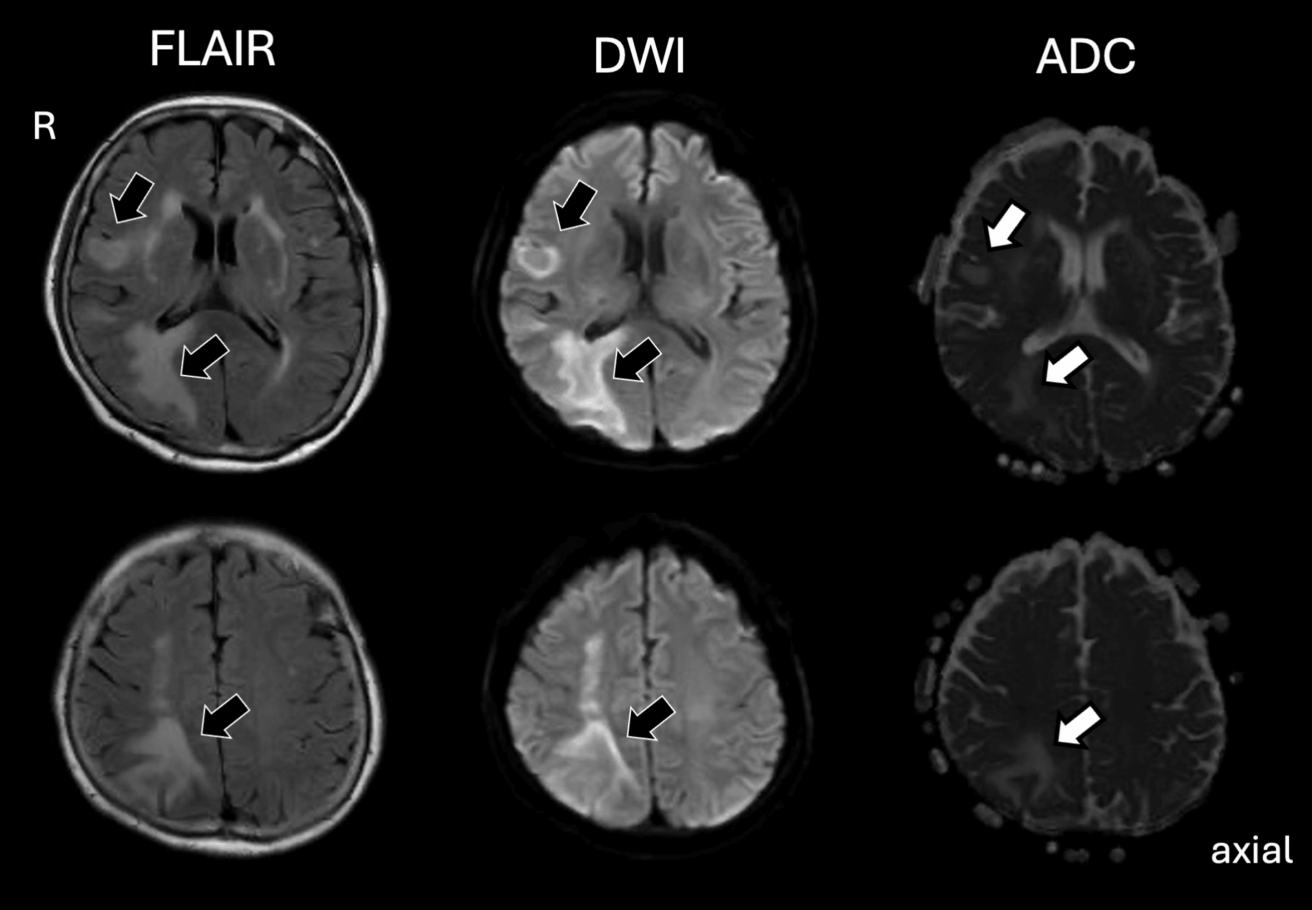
The brain findings of the onset of neurological symptoms of progressive multifocal leukoencephalopathy (PML). Brain MRI with FLAIR sequences and DWI imaging showed high intensity and subtly enlarged lesions in the right parietotemporal and frontal subcortical regions (left and middle). The lesions showed high or iso intensity on apparent diffusion coefficient (ADC) (right). FLAIR: fluid-attenuated inversion recovery; DWI: diffusion-weight image; ADC: apparent diffusion coefficient.
